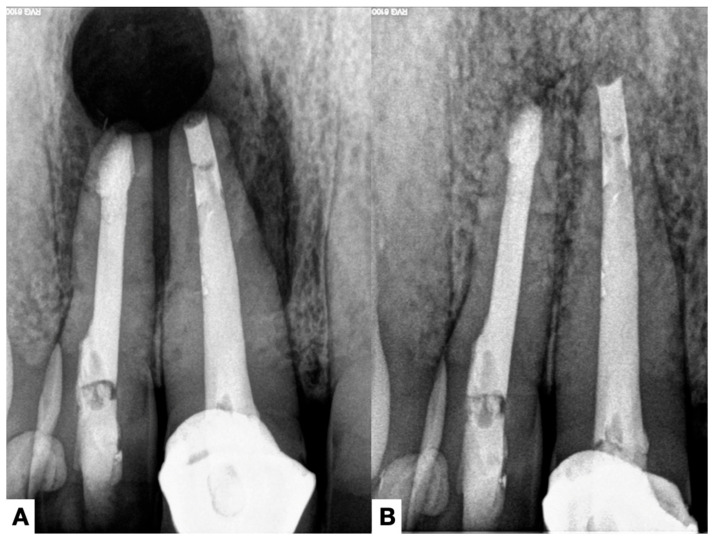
(A) Radiograph immediately after the microsurgery and (B) one-year recall periapical radiograph showing almost complete healing of the lesion.
